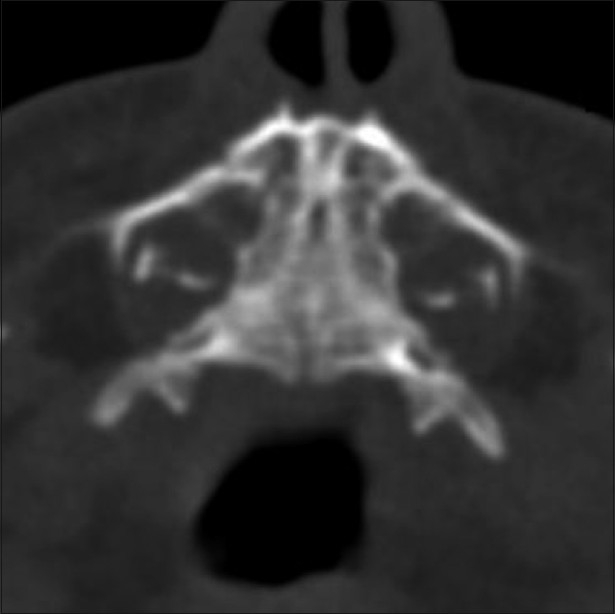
Axial CT scan shows a triangular hard palate
